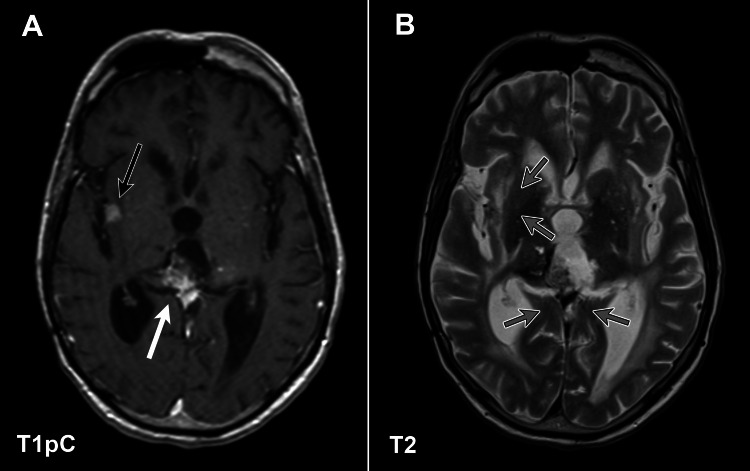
T1 post-contrast (A) and T2 MRI imaging (B) of a recurrent pineal gland glioblastoma with minimal progression at 263 weeks post-biopsy. The pineal gland demonstrates stable post-contrast enhancement (white arrow), while there was mild growth of the enhancing lesion of the right insular cortex to 9 mm (black arrow). The gray arrows demonstrate a lack of edema in the parenchyma surrounding both the primary and right insular cortex lesions on T2 MRI.
